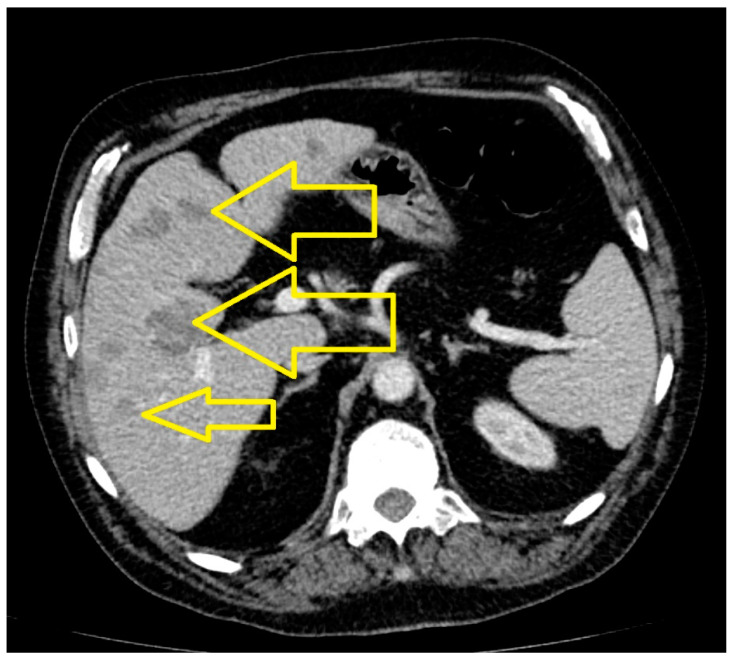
Abdominal CT (June 2024): Liver with slightly increased dimensions, with multiple liver metastases and with a diameter of up to 35 mm.
